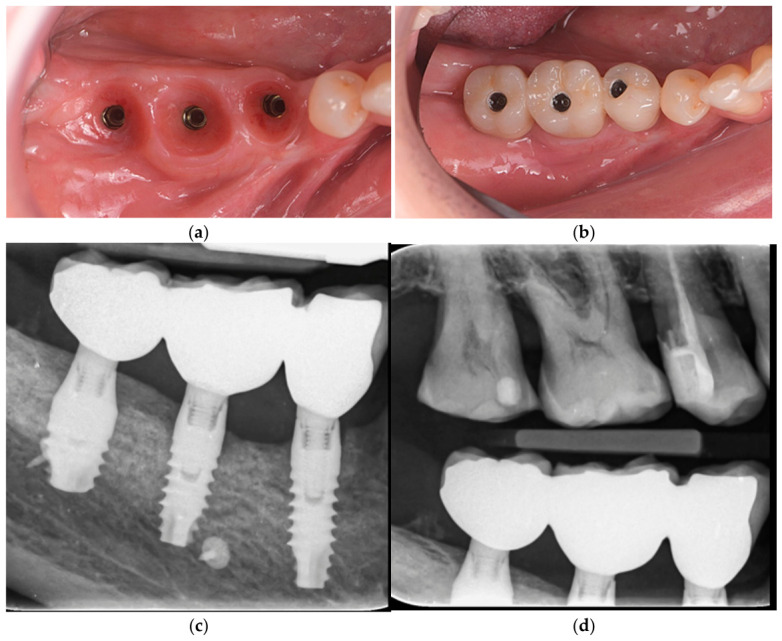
Representative prosthetic rehabilitation outcomes. (a) Peri-implant soft tissue maturation at prosthetic stage; (b) Occlusal view of the screw-retained implant-supported restorations; (c,d) Radiographic confirmation of implant positioning and marginal adaptation.
